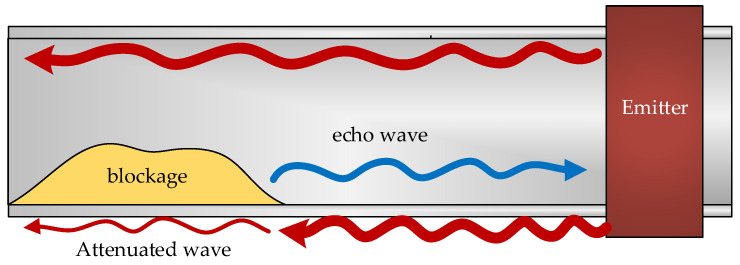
Blockage detection. Once the ultrasonic wave reaches the blockage, it produces an echo that can be used to determine its position, but the wave continues, with certain attenuation, in the same direction. The attenuation introduced by the blockage depends on the material.
